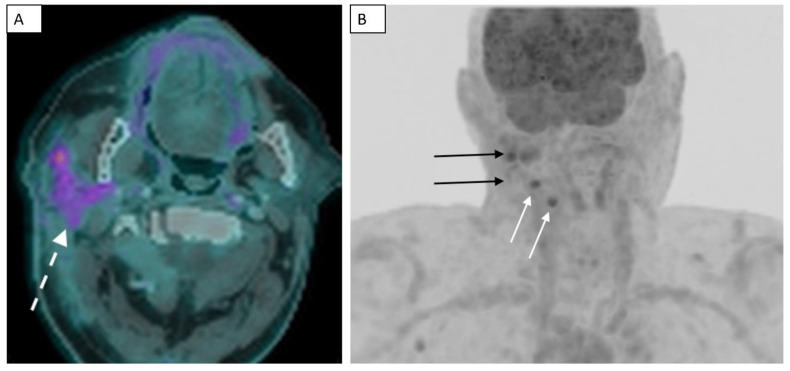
Fused PET–CT scan demonstrates FDG uptake in the infiltrative primary lesion in the right parotid gland (dashed white arrow) (A). The 3D MIP image from the PET–CT scan shows the asymmetric ill-defined FDG uptake in the parotid gland and adjacent soft tissues of the right neck (solid black arrows), as well as FDG uptake in two ipsilateral lymph nodes (solid white arrows) (B).
